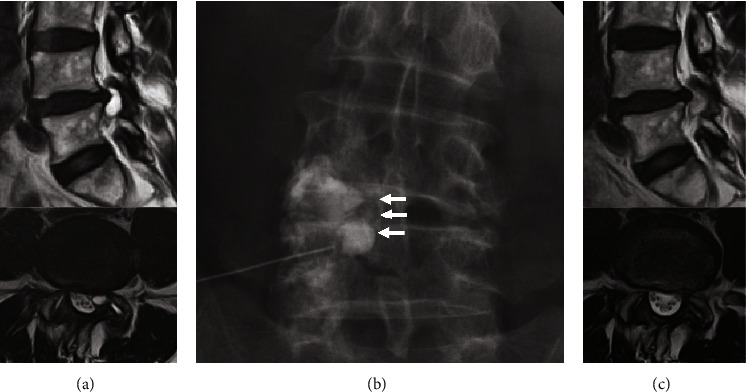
The case with successful rupture of the cyst: (a) pretreatment MRI, (b) successful rupture of the cyst (arrow: leak of contrast agent), (c) posttreatment MRI. MRI, magnetic resonance imaging.
